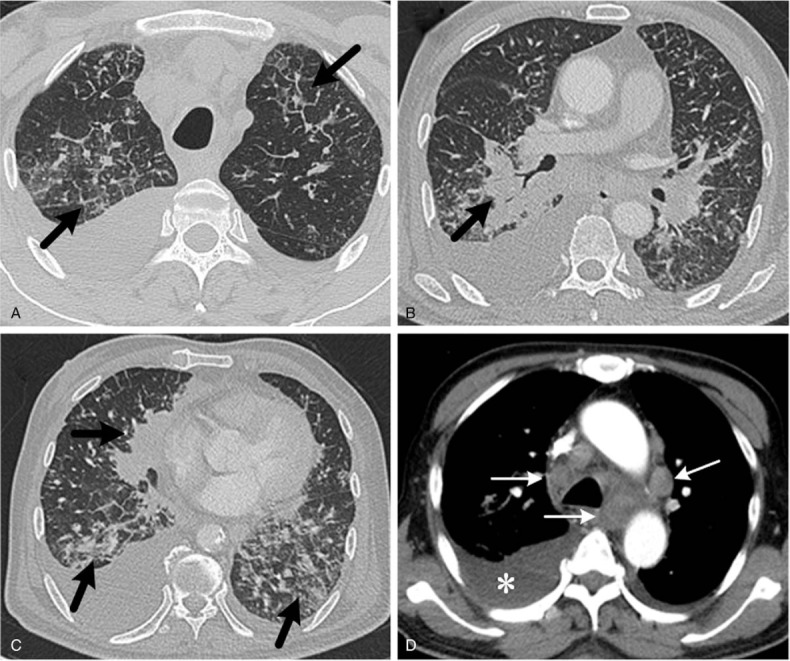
Chest high-resolution computed tomography (HRCT) shows the bilateral areas of diffusely thickened interlobular septa, the thickening of bronchovascular bundles (A–C, thick arrows), the presence of enlarged mediastinal nodes (D, thick arrows), and pleural effusion (D, asterisk). All these features indicate progression of disease.
